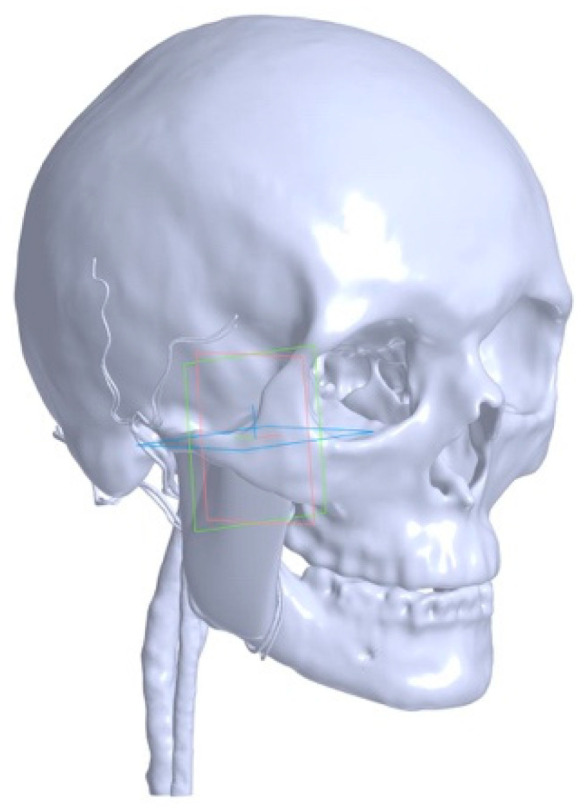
Solid-state NURBS model derived from CT data and the BodyParts3D anatomical atlas. The model includes the skull, mandible, temporomandibular joint (TMJ), masticatory muscles (masseter, medial pterygoid, lateral pterygoid), external carotid artery, internal jugular vein, and the pterygoid venous plexus (PVP).
